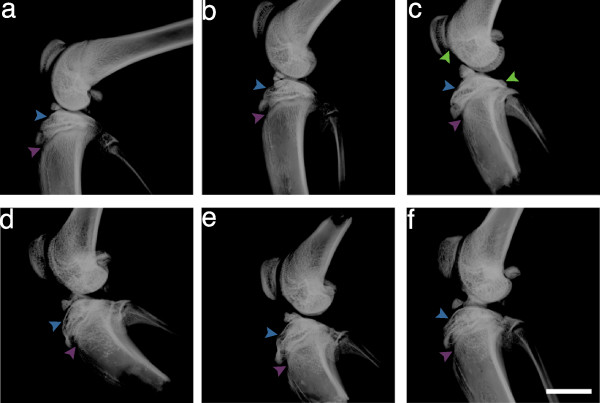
Antagonist treatment improves radiological indices of OA: X-ray results. (a) No surgery and saline treatment; (b) sham surgery and saline treatment; (c) ACLT and saline treatment; (d) ACLT and BQ-123 treatment; (e) ACLT and R-954 treatment; (f) ACLT and BQ-123+R-954 dual treatment. Blue arrows indicate tibial plateau, purple arrows indicate subchondral bone, and green arrows indicate osteophytes. Sagittal views. Scale bar, 1 cm.
